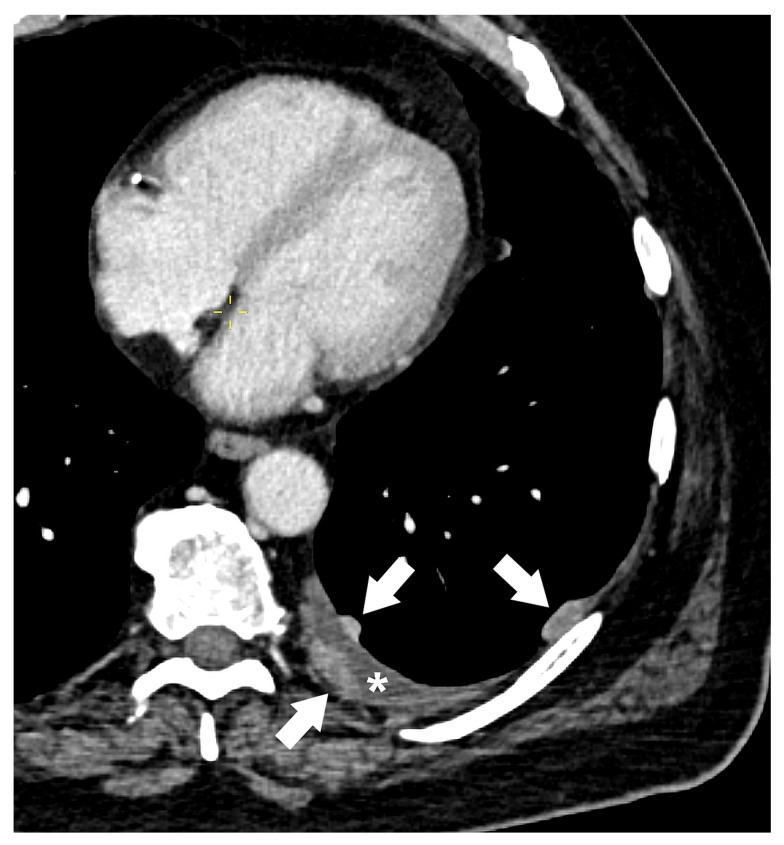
M1a category: Contrast-enhanced CT image showing left-sided pleural enhancing nodules (arrows) with ipsilateral pleural effusion (asterisk), indicating pleural metastases (M1a).
